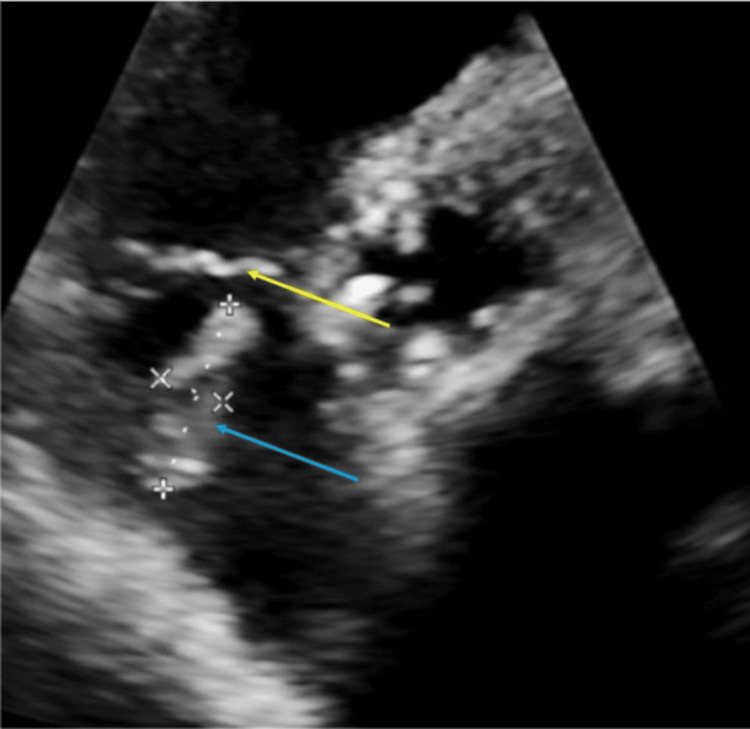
Basal parasternal short axis view from the transthoracic echocardiogram demonstrating the suspected vegetation (blue arrow) and tricuspid valve (yellow). Right ventricle implantable cardiac defibrillator lead not visible in image. Echocardiogram obtained on day three of admission.
